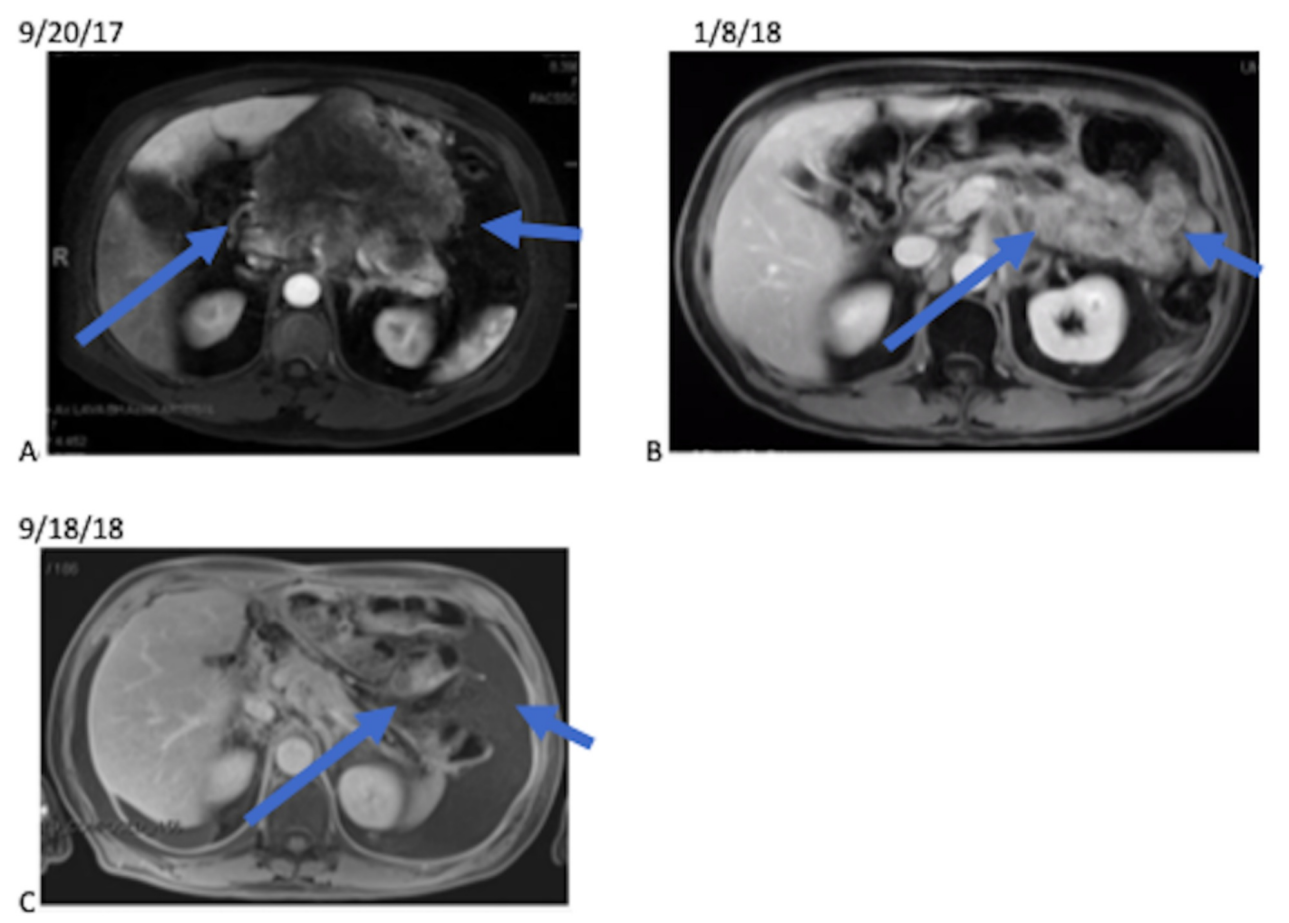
Magnetic Resonance Images Imaging from pre- and post-treatment. A) A T1 post-contrast MRI shows a large gastric mass with heterogeneous borders, which is 16.7 cm x 14.1 cm (blue arrows). B) A T1 post-contrast MRI after chemoradiation shows an improvement in gastric mass (blue arrows) with the ability to visualize bowel. C) A T1 post-contrast MRI after surgery showing no evidence of disease (blue arrows).
